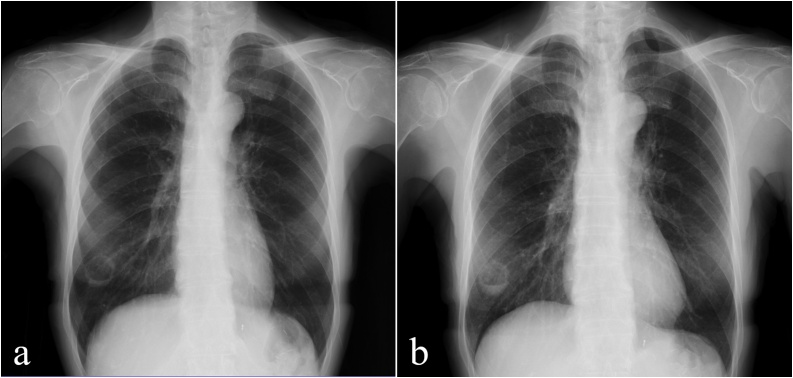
Chest X-ray findings. a: 10 days after the medication. Fluid inside disappeared. b: after 4weeks without antibiotics. The liquid in the cavity reappeared.
